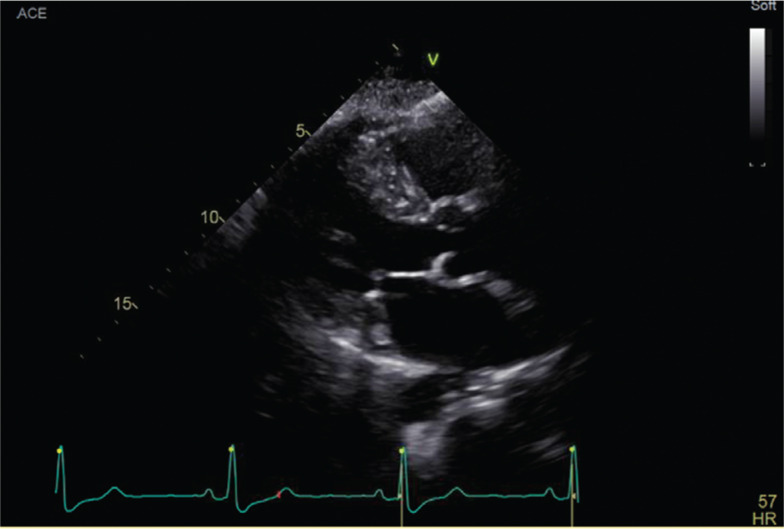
Echocardiogram after device implant.
